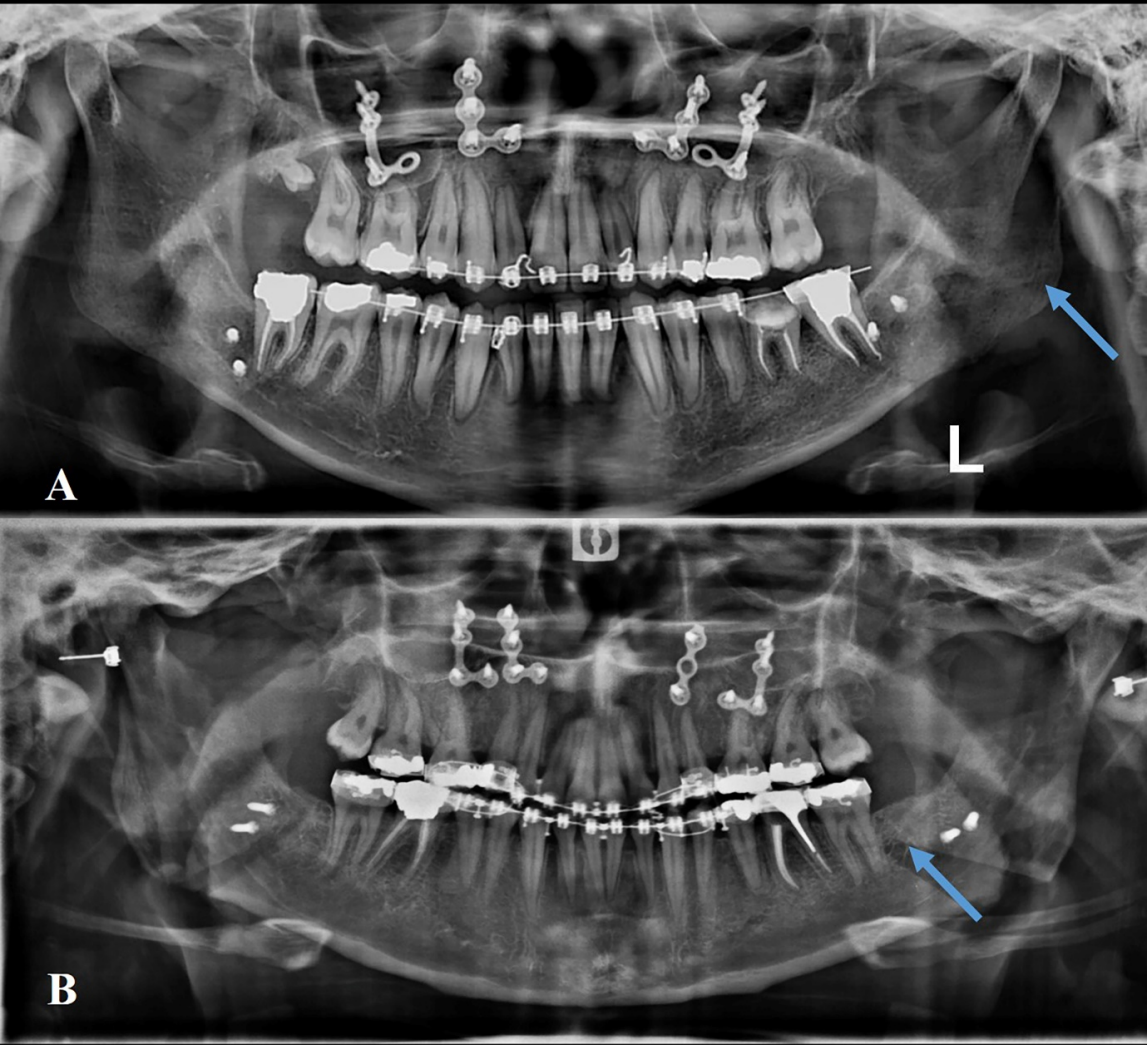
The radiographic view of bad split in the Hunsuck (A) and Dal Pont (b) techniques
